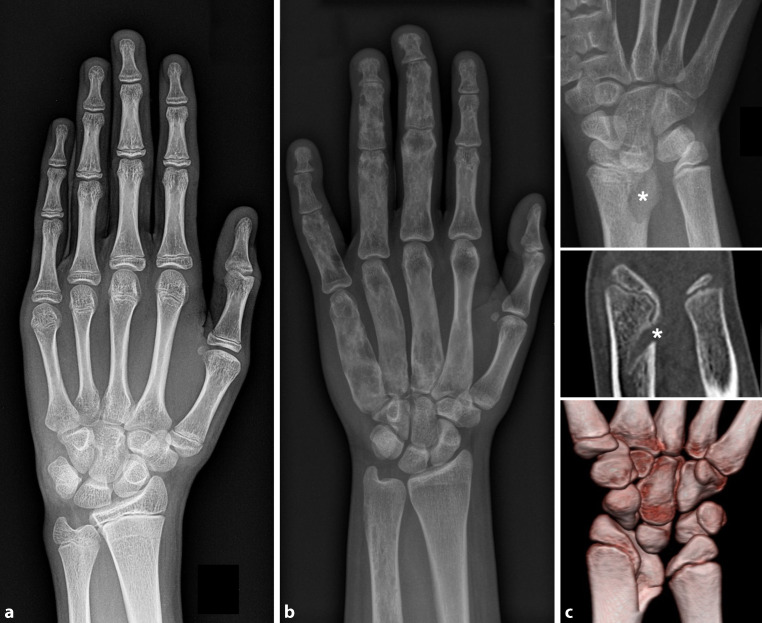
Conventional hand radiographs. a Healthy 15-year-old girl. b Polyostotic fibrous dysplasia in McCune–Albright syndrome. c Madelung deformity in a girl with SHOX-deficiency (*top*: radiographs; *middle* and *bottom*: computed tomography with 3D reconstruction). *Asterisks* highlight the bony deformities of the distal radius (with ulnar and volar tilting, decreased carpal angle) and the pathologic thickening of the short radiolunate ligament (Vicker’s ligament). There is secondary triangularization of the lunate
